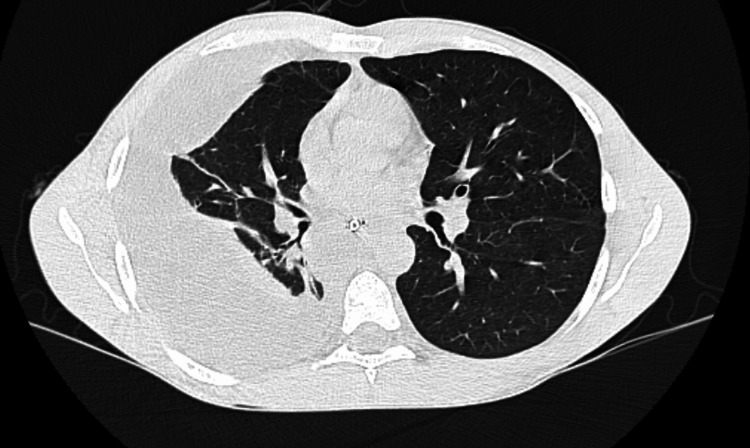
Follow-up axial chest CT image demonstrating complete resolution of the pneumomediastinum, with associated right-sided pleural effusion.
